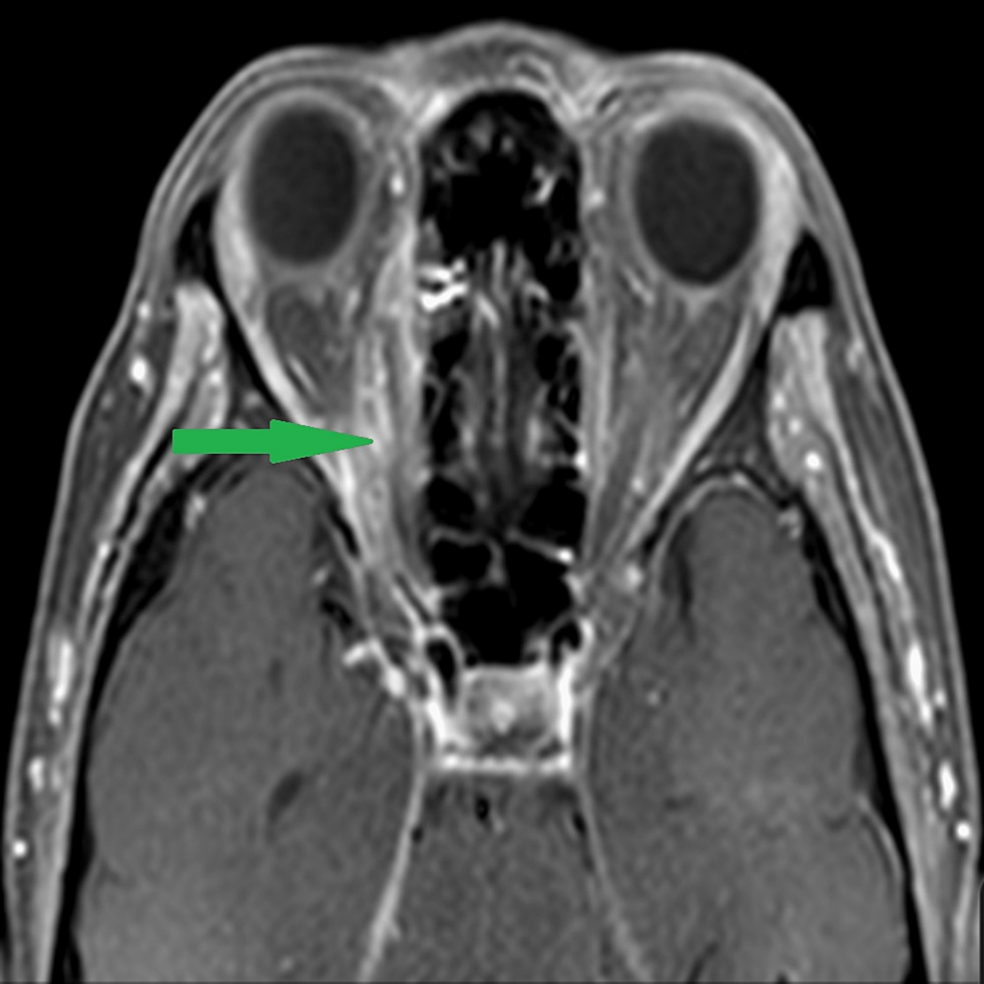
Axial cut of contrast MRI of the brain and orbit showing ill-defined lesion at the right orbital apex at the superomedial margin of the optic nerve with the presence of enhancement of adjacent intraconal and extraconal fat (green arrow) MRI: magnetic resonance imaging
